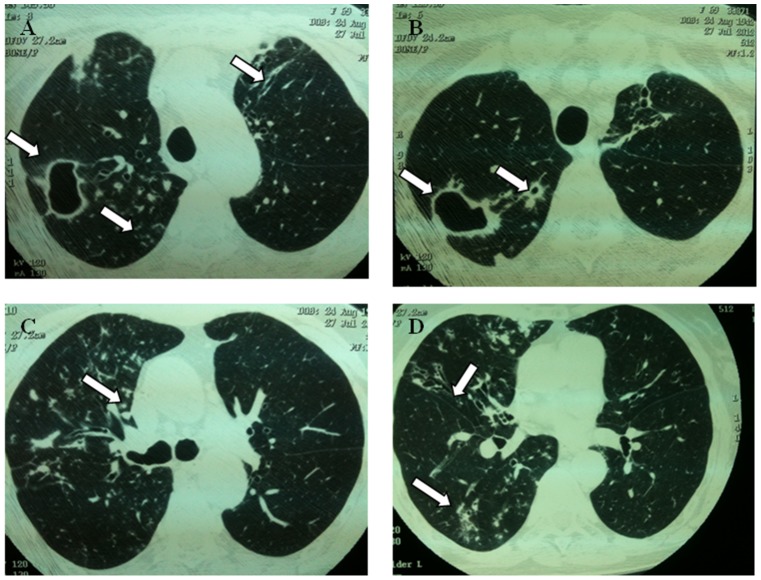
Representative image showing lung damage in a patient with nontuberculous mycobacterial diseases. A 69-years-old woman with *Mycobacterium abscessus* pulmonary disease. (A and B) High resolution computed tomography (HRCT) of the chest obtained at level of upper lobes showing multiples cavities in the right upper lobe and centrilobular nodules. It is also possible to observe bronchiectasis in left upper lobe (arrow). (C) Tree-in-bud pattern. (D) Presence of bronchiectasis in middle lobe. Also note centrilobular nodules at right lower lobe.
